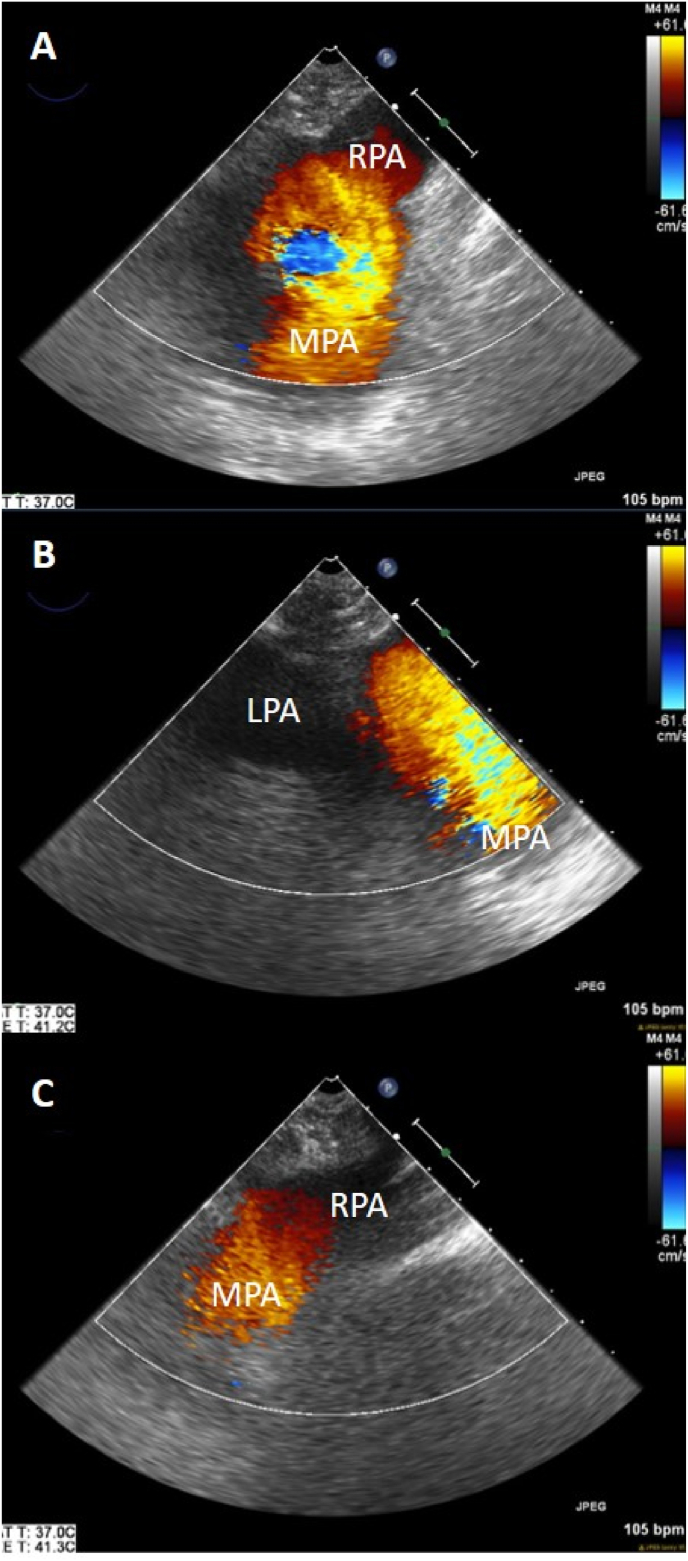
Intraoperative transesophageal echocardiogram images with color flow doppler of the main pulmonary artery (MPA), left pulmonary artery (LPA) and right pulmonary artery (RPA) prior to surgical pulmonary embolectomy. The images reveal significant flow acceleration across a dilated MPA (Panel A) and minimal to no antegrade flow into the LPA (panel B) and RPA (panel C). (For interpretation of the references to color in this figure legend, the reader is referred to the Web version of this article.)
